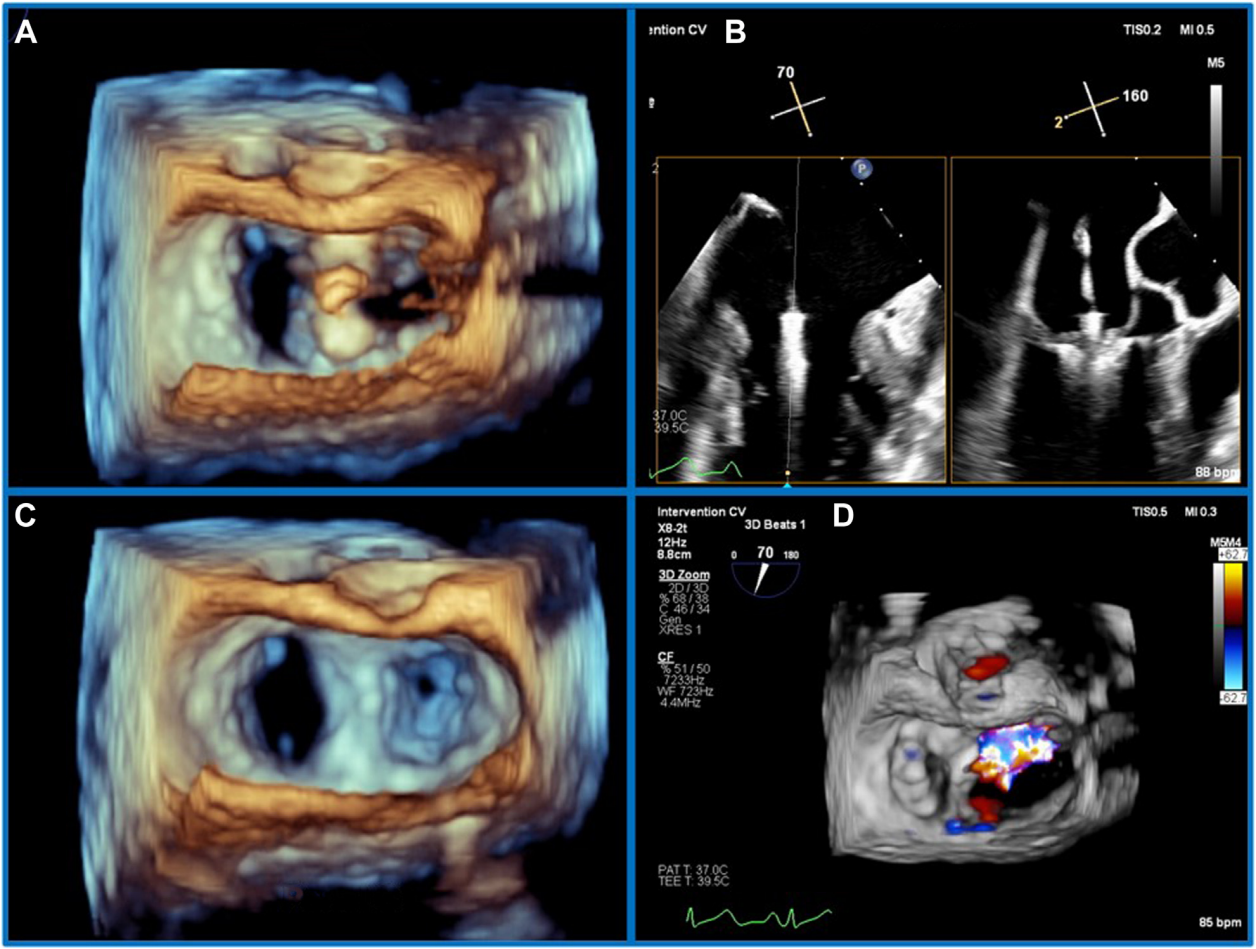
Insertion of first M-TEER device. Three-dimensional TEE, midesophageal, volume-rendered reconstruction en face MV view, demonstrates optimal orientation of the device over central A_2_P_2_(A) and confirms a broad tissue bridge after release (C). (B) Two-dimensional TEE, simultaneous long-axis display from the bicommissural view, demonstrates optimal grasping view with ideal grasping position of the device. (D) Three-dimensional TEE, midesophageal, volume-rendered reconstruction en face MV view with color flow Doppler, demonstrates residual severe MR medial to the device.
